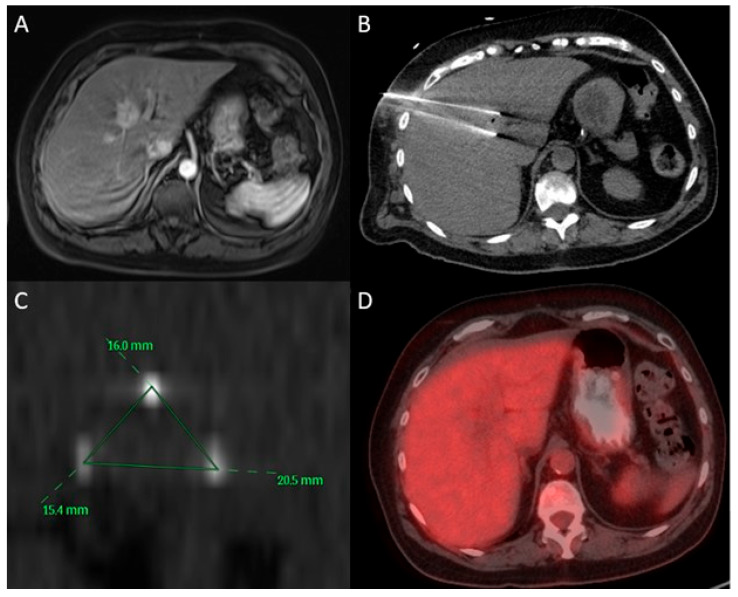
A 67-year-old female with a history of bilateral breast cancer (right invasive lobular carcinoma, ER/PR positive, HER2 negative), diagnosed in 2015, status post mastectomy and adjuvant chemotherapy. She developed bone metastases in 2021 and is being treated with Xeloda and Fulvestrant. (A) Abdominal MRI demonstrates an enhancing, diffusion-restricting mass within segment 8/5, partially extending into 4a/4b, measuring approximately 3.4 × 2.4 cm. (B,C) Intraprocedural CT showing intermittent placement of three 17-gauge NanoKnife IRE ablation needles. (D) Follow-up PET-CT at 3 months reveals a calcified granuloma in the right hepatic lobe with no evidence of focal FDG-avid lesions in the liver.
